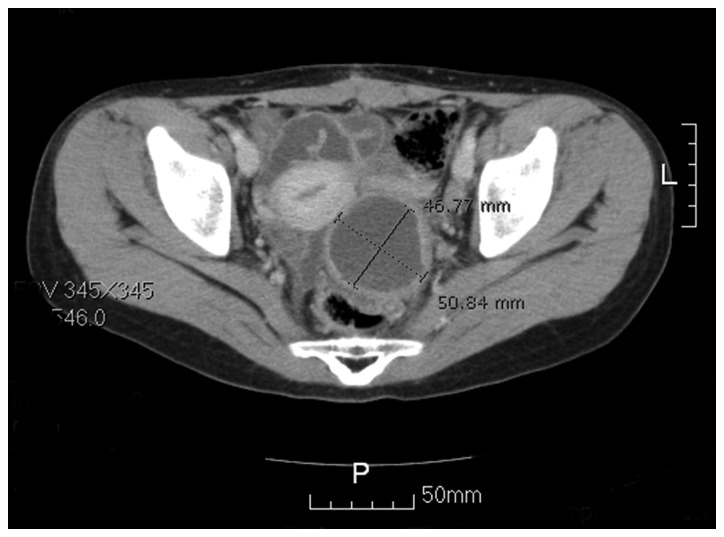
Computed tomography scan of the tumor. The size of the mass was 4.6×5.0 cm, which was significantly reduced compared with Fig. 1.
